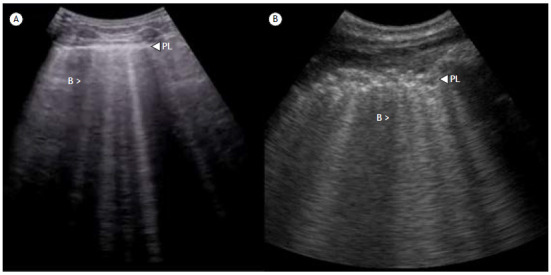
a) Multiple B-lines (B) can be seen as vertical, hyperechoic lines originating from the pleural line (PL) and stretching all the way to the bottom of the two-dimensional brightness (B)-mode image. b) Idiopathic pulmonary fibrosis: thoracic ultrasound image of the lower lobe of a patient diagnosed with idiopathic pulmonary fibrosis. Multiple B-lines are present, and the PL appears severely thickened and fragmented.
